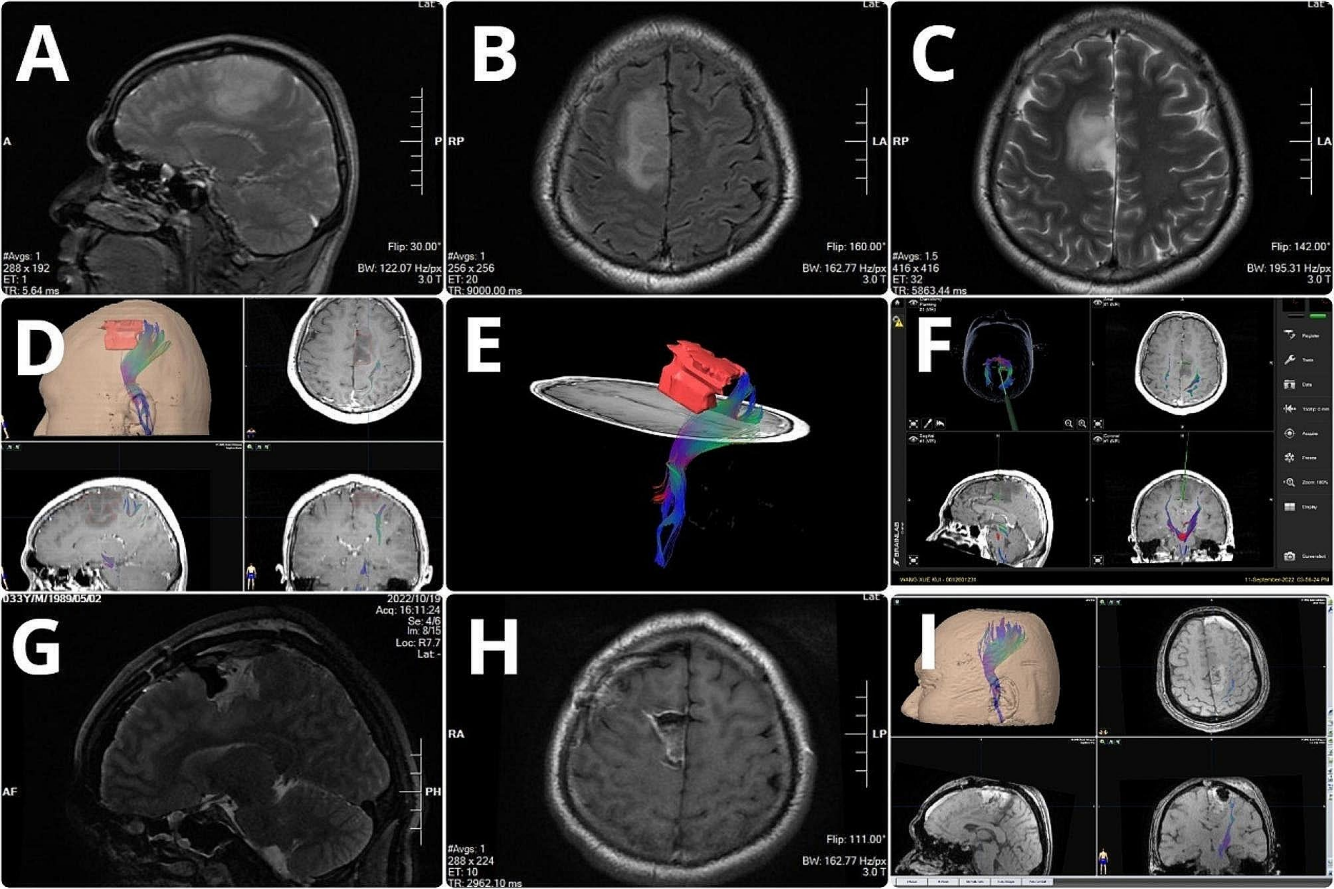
Case 1: A 33-year-old male patient. The preoperative MRI scan showed patchy long T1 and T2 signals in the right frontal lobe and subcortex (A–C). Preoperative three-dimensional reconstruction showing the spatial relationship between fiber tracts and tumor location (D, E). The preoperative plan was imported into the navigation system (F). Intraoperative MRI and intraoperative DTI three-dimensional reconstruction showed complete tumor resection and preservation of intact fiber tracts (G–I). DTI, diffusion tensor imaging; MRI, magnetic resonance imaging
